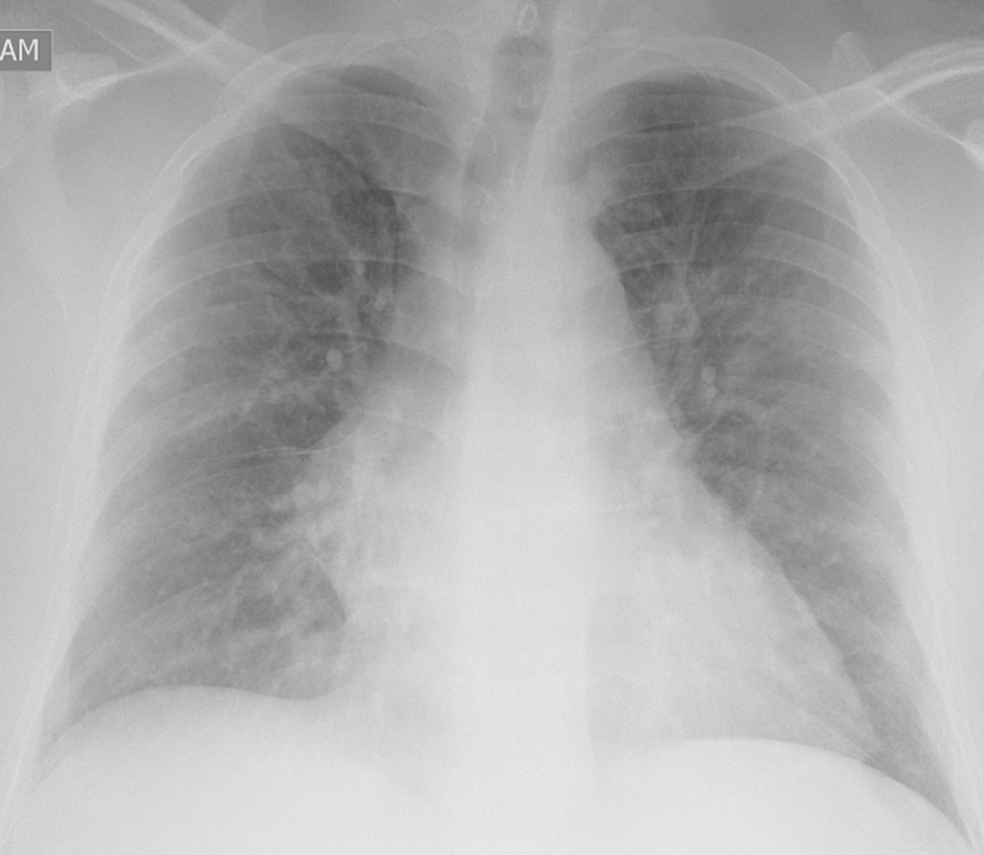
Chest X-ray revealed diffuse bilateral infiltrates, consistent of COVID-19 pictures
